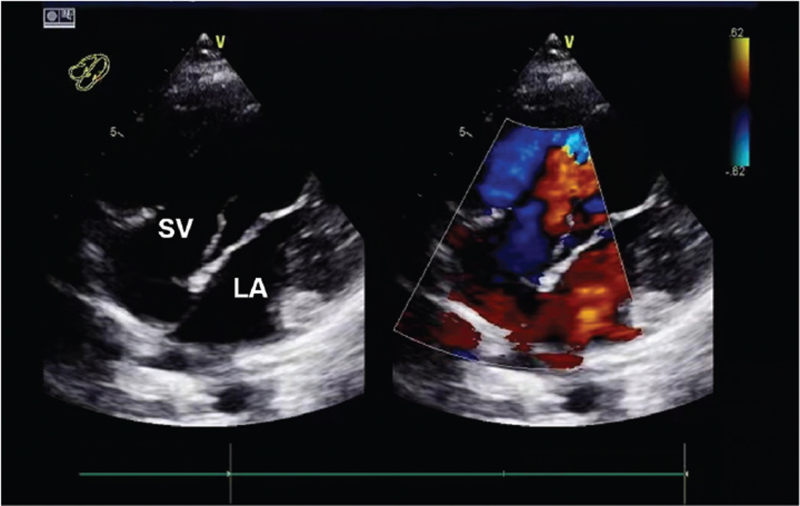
Parasternal long axis view of transthoracic echocardiography showing a single ventricle where the left ventricle is dominant. LA = left atrium, SV = single ventricle.
